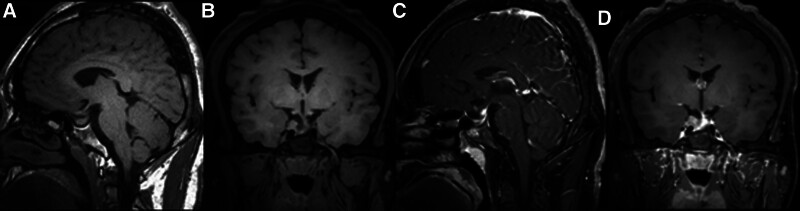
Patient MRI on July 4, 2020 (A and B:T1WI; C and D: enhanced MRI).
